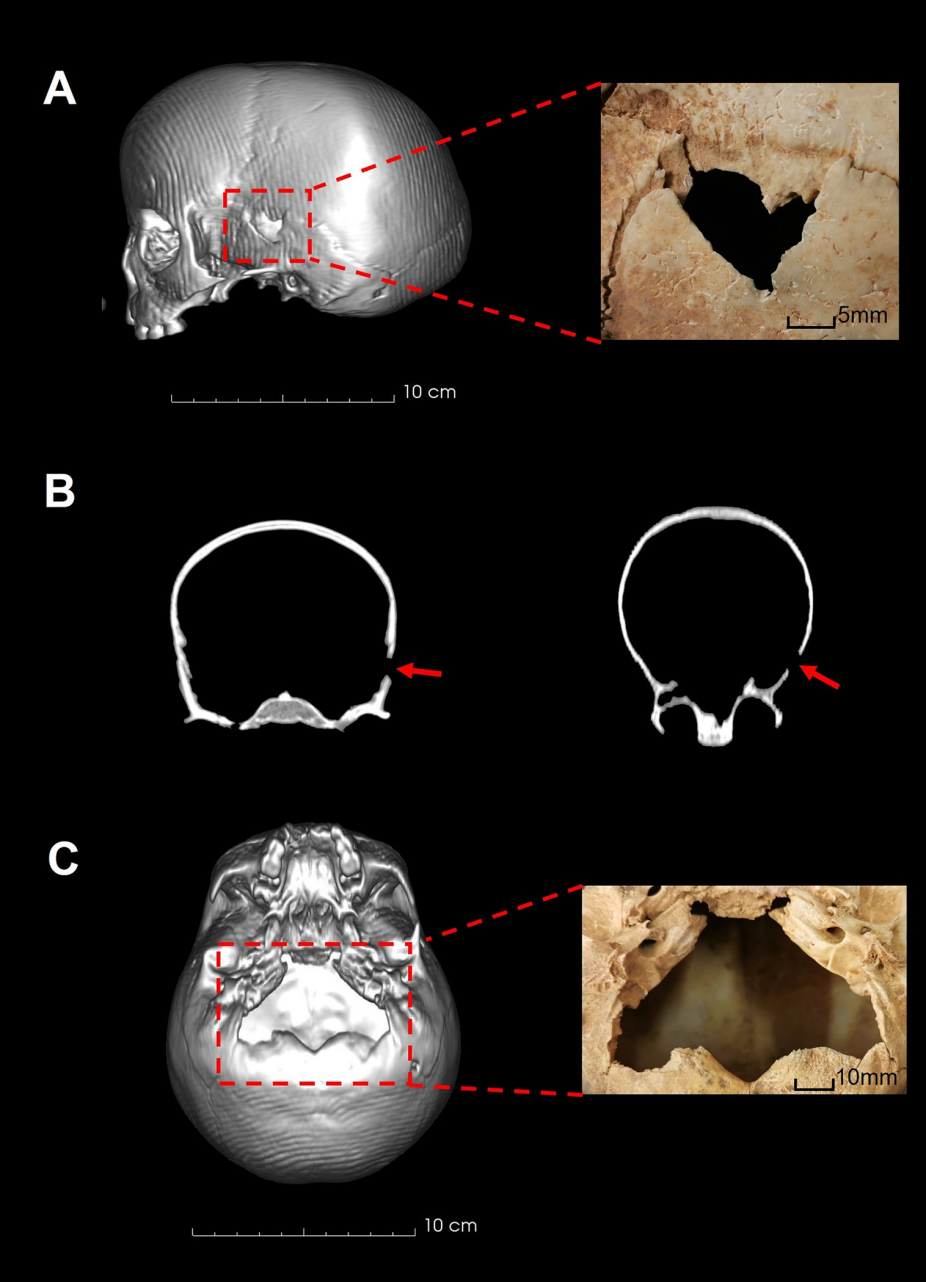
Cranial injuries on UCT 150. A: 3-Dimensional CT rendering of left cranium. Red box: Penetrating lesion to the left temporal region B: Coronal and axial cross-section CT demonstrating injury location and impact direction. C: 3-Dimensional CT rendering of inferior cranium. Red box: Penetrating lesion to the basal portion of the occiput.
